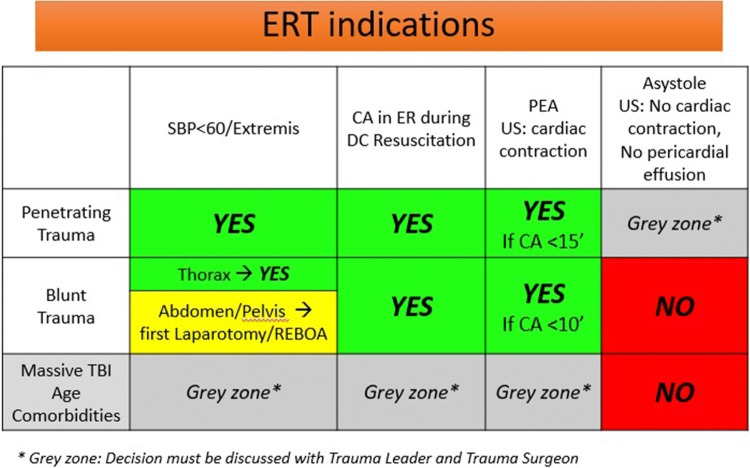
This table is daily used in the ER and it is more user friendly in the critical situations, such as during the survey of a major trauma patient. *SBP* systolic blood pressure, *CA* cardiac arrest, *ER* emergency room, *DC* damage control, *PEA* pulseless electrical activity, *US* ultrasonography, *TBI* traumatic brain injury
